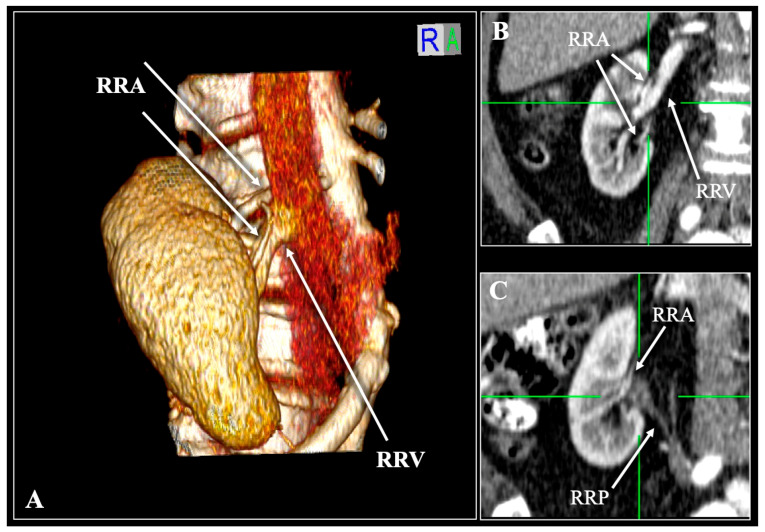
Contrast-enhanced computed tomography (CT) scan of a 58-year-old female patient demonstrating the Artery (A)—Vein (V)—Artery (A)—Pelvis (P) variation. Three-dimensional reconstruction (A) and multiplanar reconstruction with coronal (B,C) slices. A single right renal vein (RRV) and a bifurcated right renal artery (RRA) entering the hilum. RRP—right renal pelvis. R—right, L—left, S—superior, I—inferior.
